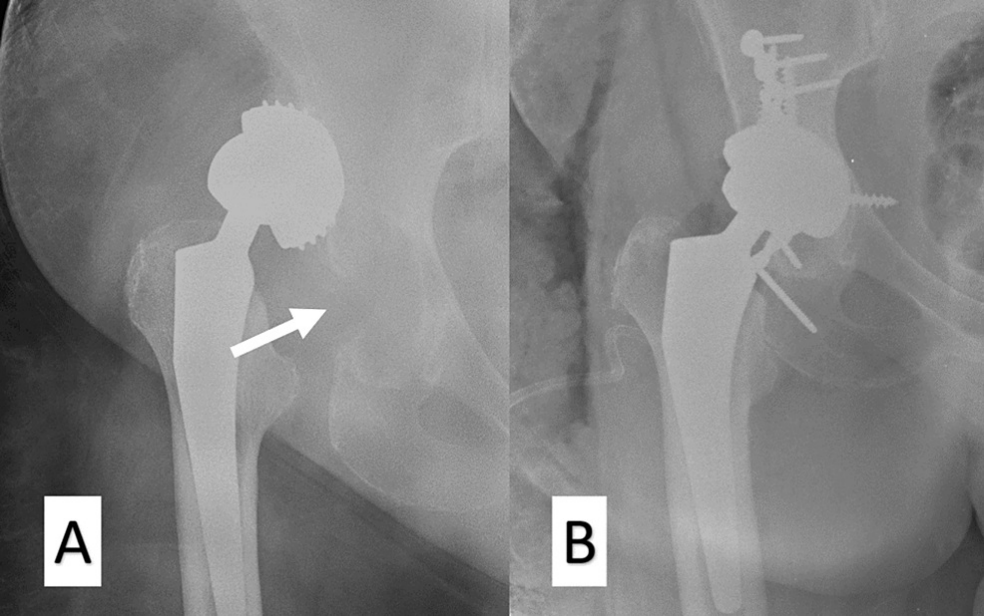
Postoperative anteroposterior X-rays of the patient A: Postoperative anteroposterior X-ray view after mobilization of the patient. A posterior wall acetabulum fracture was noted (white arrow), while the threaded cup had migrated proximally. B: Anteroposterior X-ray view after revision surgery. Open reduction and internal fixation and placement of a press-fit multi-hole cup were done
